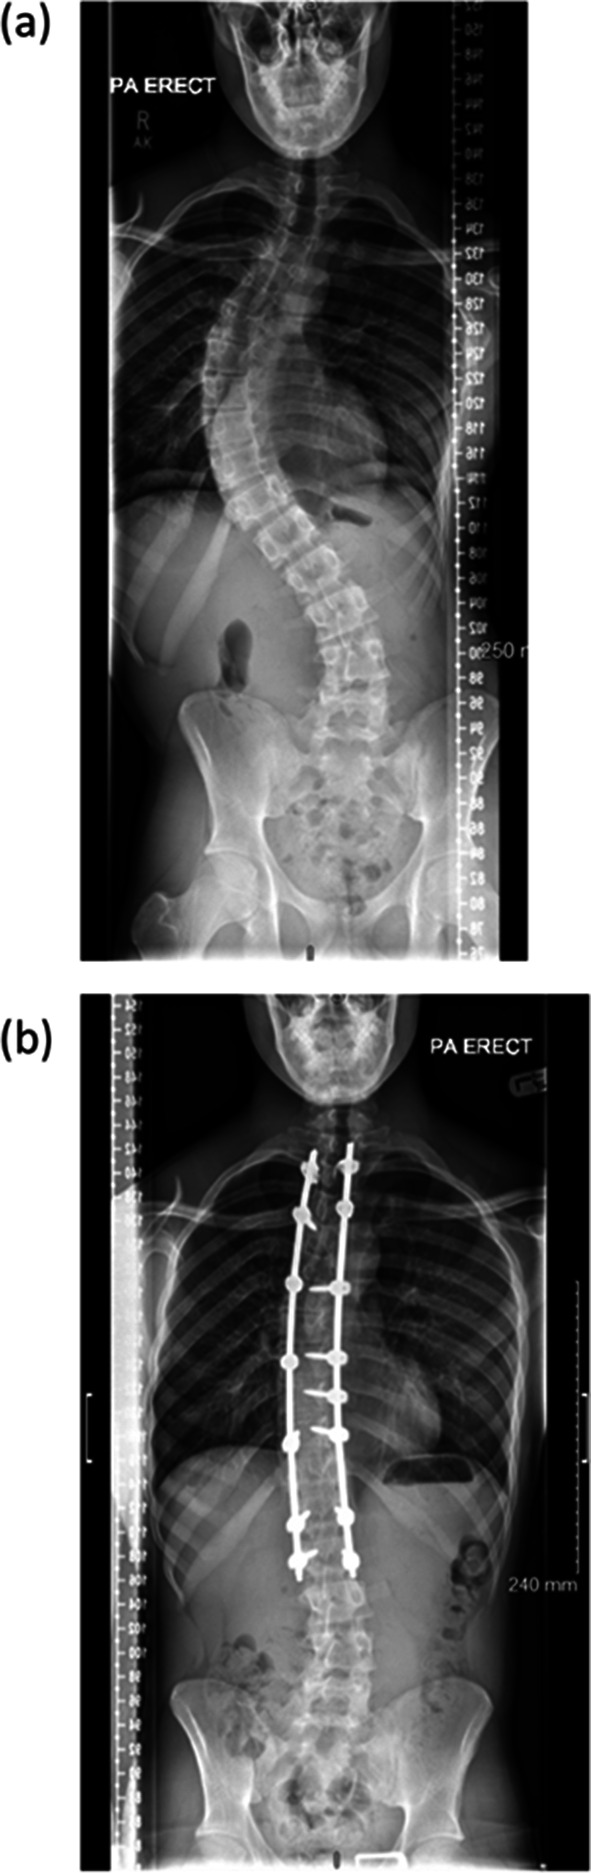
Radiographic images before and after the posterior-only instrumented fusion. a Preoperative standing antero-posterior radiograph. b postoperative standing antero-posterior radiograph
